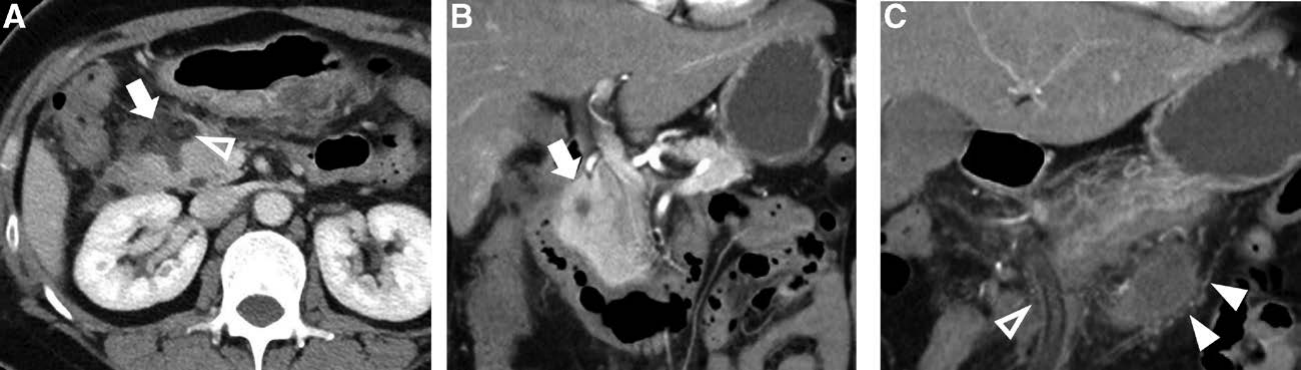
Post-operative contrast-enhanced abdominal-pelvic CT (POD 7). (a) Axial portal venous phase image. Focal parenchyma defect (white arrow) was revealed where the tumor originally situated, with effusion and draining tube in situ (hollow arrowhead). (b and c) Coronal portal venous phase image showing the parenchyma defect (white arrow) as the result of tumor enucleation. The draining tube was in situ (hollow arrowhead), and there was fluid accumulation with peripheral fat stranding on the caudal side of the pancreas, indicating post-operative fistula. CT = computed tomography, POD = postoperative day.
